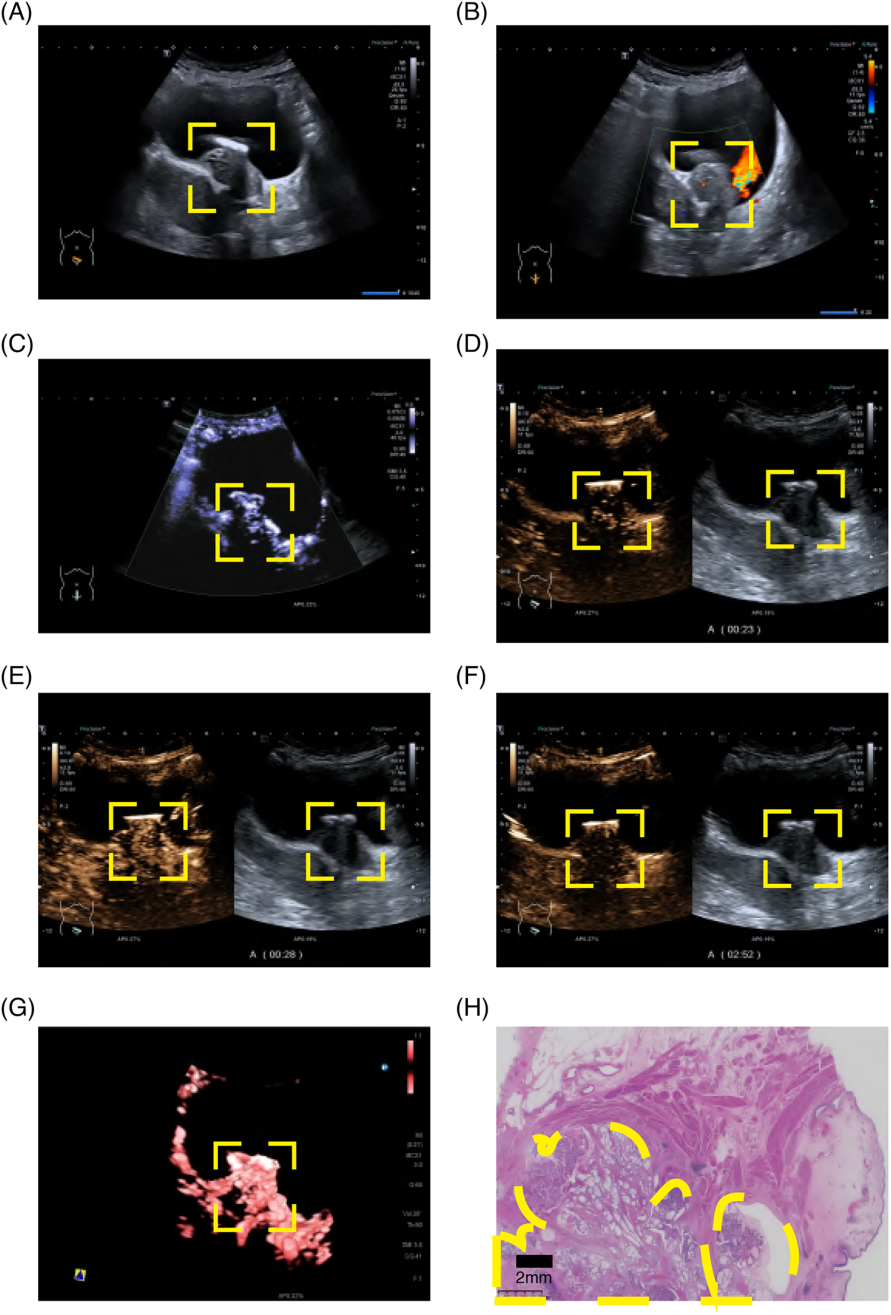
Ultrasound characteristics of muscle invasive bladder cancer patients and pathology status. (A) gray‐scale ultrasound; (B) color doppler flow imaging; (C) contrast harmonic imaging + superb micro vascular imaging; (D) contrast harmonic imaging in 23 s just after the lesion begins to strengthen; (E) contrast harmonic imaging in 28 s almost before the lesion reaches the peak; (F) contrast harmonic imaging in 172 s after contrast material has washed out; (G) contrast harmonic imaging + superb micro vascular imaging + smart 3D; (H) pathology imaging. The yellow boxes highlight the lesions.
